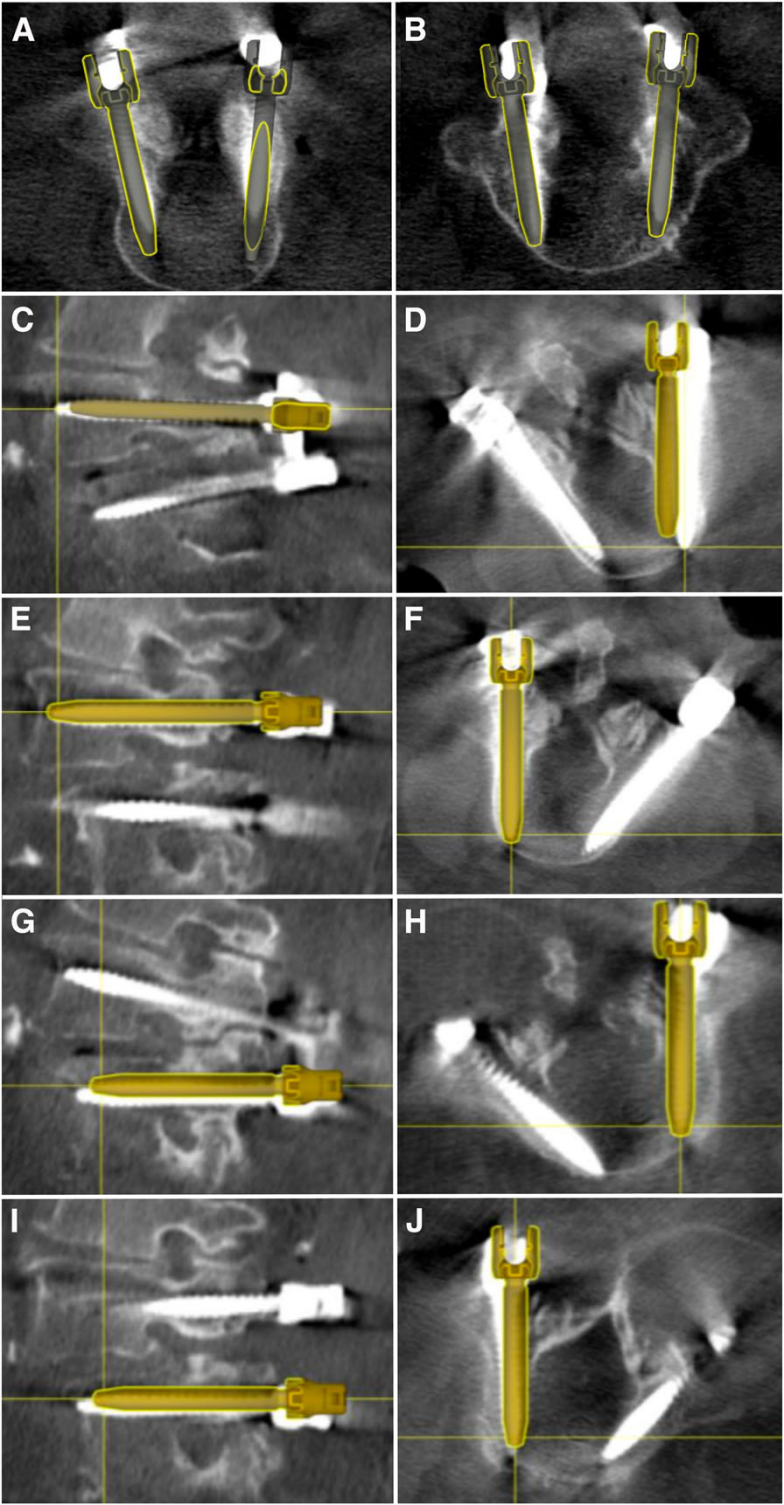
Planned versus placed screw trajectories in ExcelsiusGPS® assisted lumbar fusion. a Axial CT overlay reconstruction with placed screw and pre-planned 3D trajectory in Patient 1, who underwent L4 and (b) L5 instrumentation. c-d Sagittal and axial CT overlay reconstruction in Patient 2 demonstrating deviation of actual right L4 screw placement from pre-planned trajectory, but without medial or lateral cortical breach. e-f Left L4 screw placement with mild deviation from pre-planned trajectory on axial and sagittal projections. g-j Left and right L5 screw placement in Patient 2 demonstrating minimal deviation from planned trajectory
